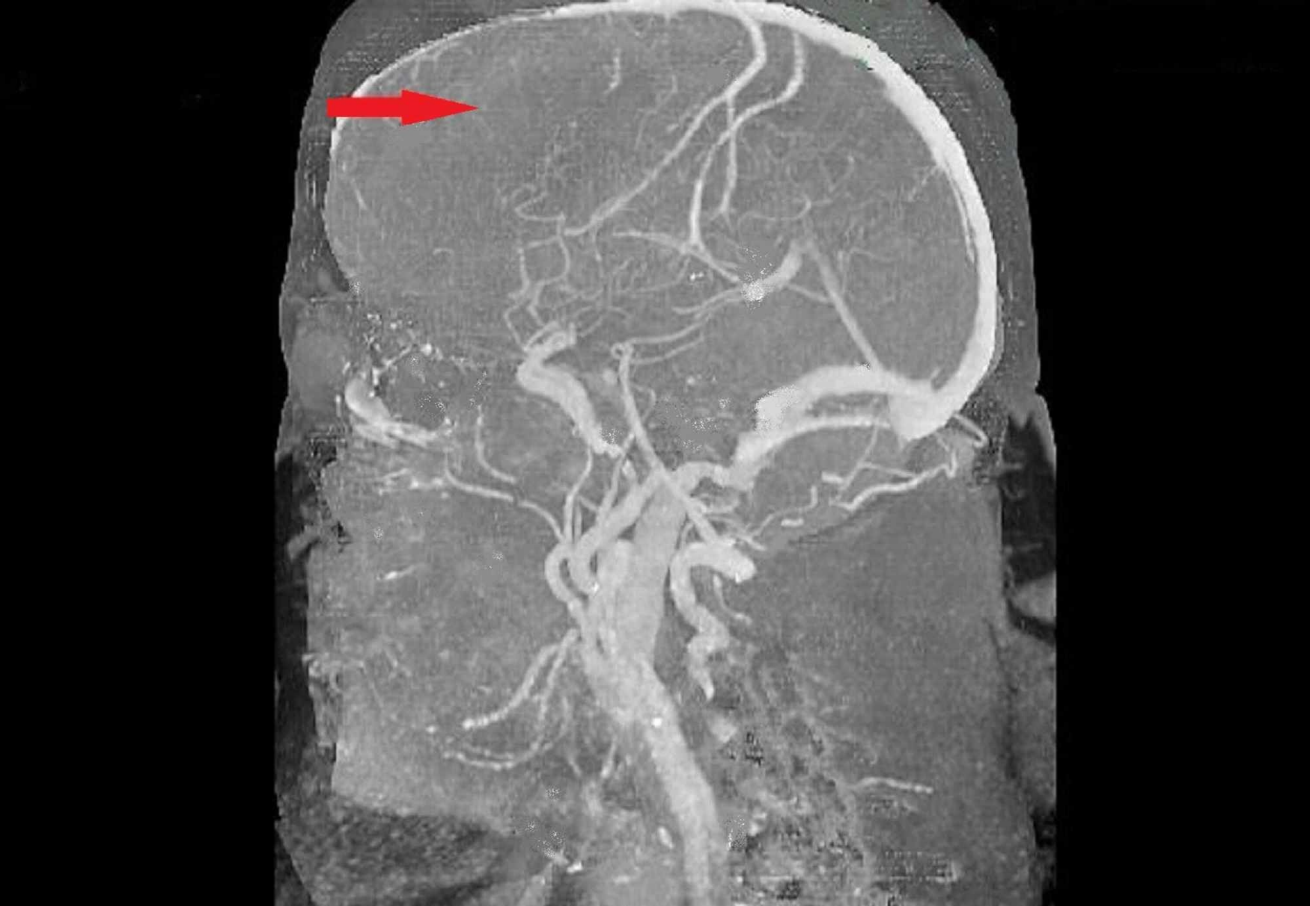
Computed tomography angiography shows occlusion of the bilateral anterior cerebral arteries (red arrow)
